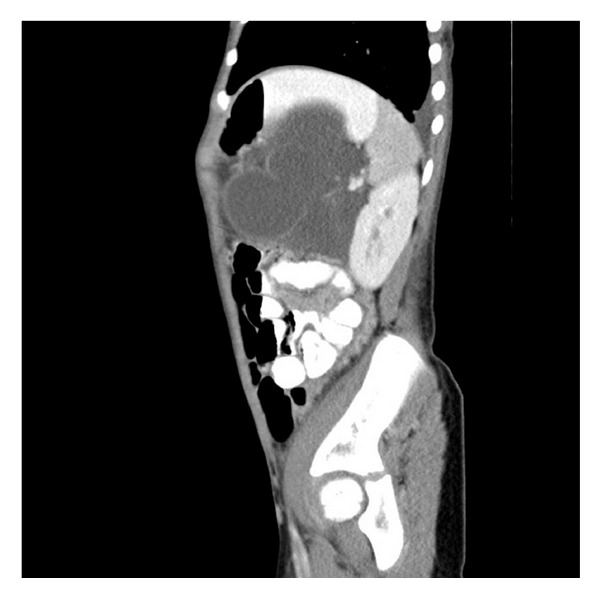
Contrast-enhanced CT sagital scan shows that retroperitoneal lobulated-septated cystic mass cannot be distinguished from spleen, pancreas, and stomach. Splenic vein and artery borders are in the cystic mass also.
